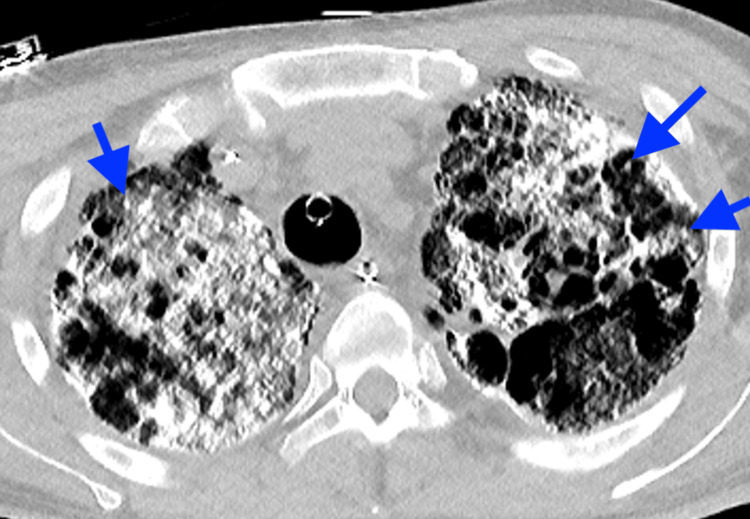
High-resolution axial chest CT showing diffuse, coalescing calcified micronodules (blue arrows) in both lungs, with subpleural and peri-bronchial distribution, interspersed with ground glass opacities
